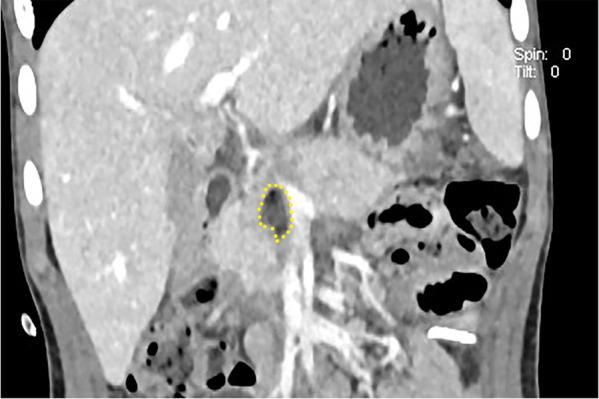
(Case 5): follow-up CT scan 9 days postoperatively shows a small amount of localized fluid collection around the anastomosis (yellow circle).
